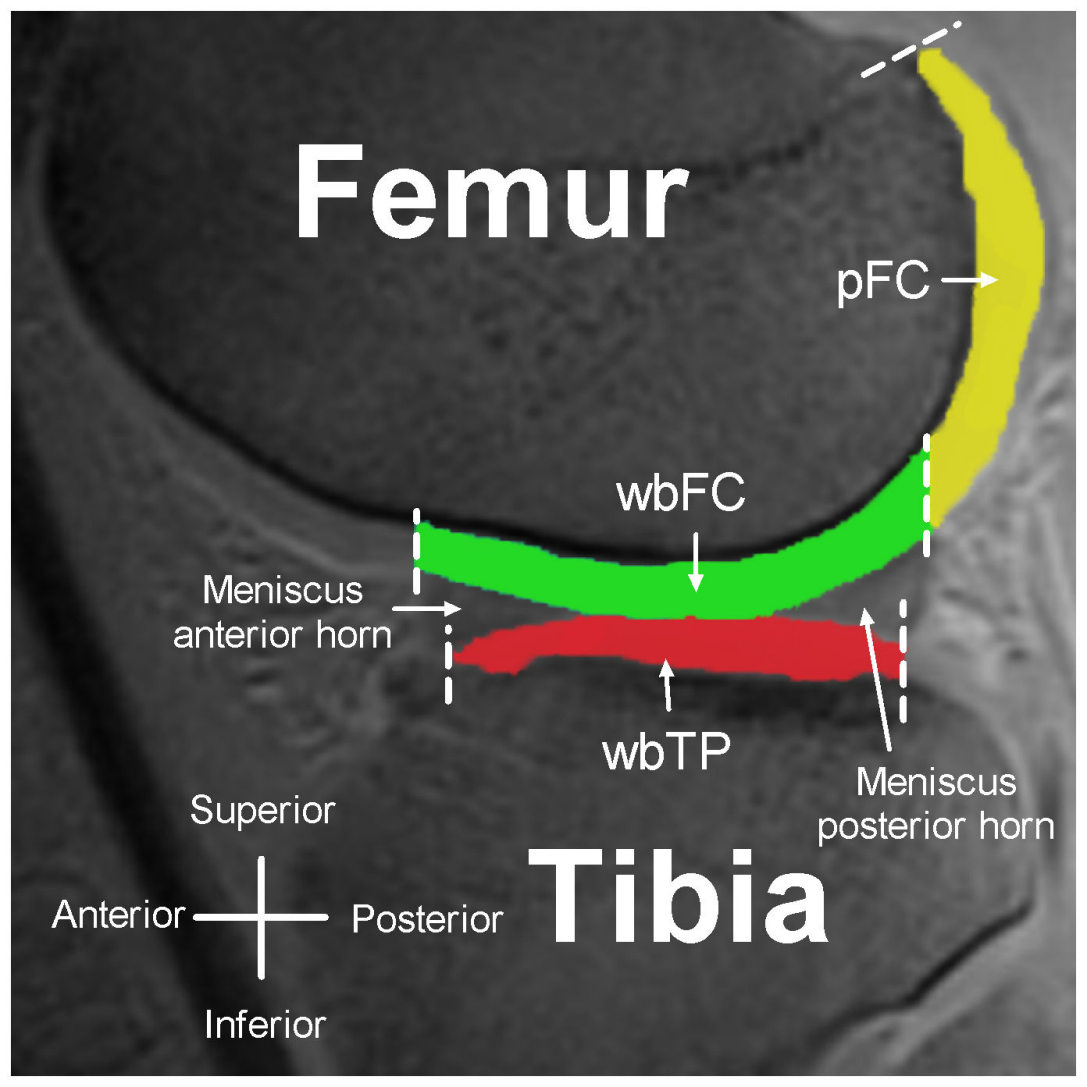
Cartilage regions* analyzed using dGEMRIC. Central sagittal MR image through the lateral tibiofemoral joint. The three anatomical cartilage ROIs which were drawn and analyzed on three consecutive images in each compartment of the tibiofemoral joint are shown. wbFC (green): weight-bearing cartilage of the femoral condyle. pFC (yellow): posterior non weight-bearing cartilage of the femoral condyle. wbTP (red): weight-bearing cartilage of the tibial plateau.
